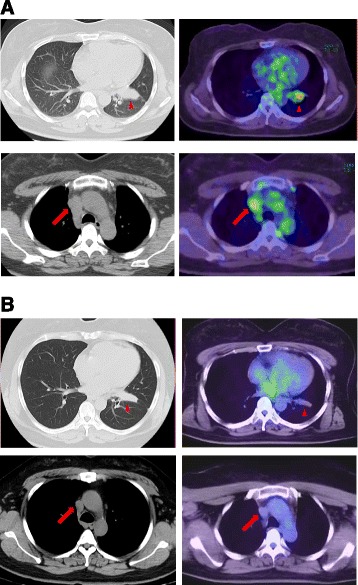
Chest CT and PET-CT images before and after crizotinib treatment. (A) Chest CT and PET-CT before treatment revealing a large primary lung mass (*arrowhead*) and multiple lymph node metastases affecting mediastinal and bilateral hilar lymph nodes (*arrow*). (B) Chest CT and PET-CT 6 months after initiation of crizotinib therapy showing significantly decreased tumor and no FDG accumulation in the primary and other lesions.
